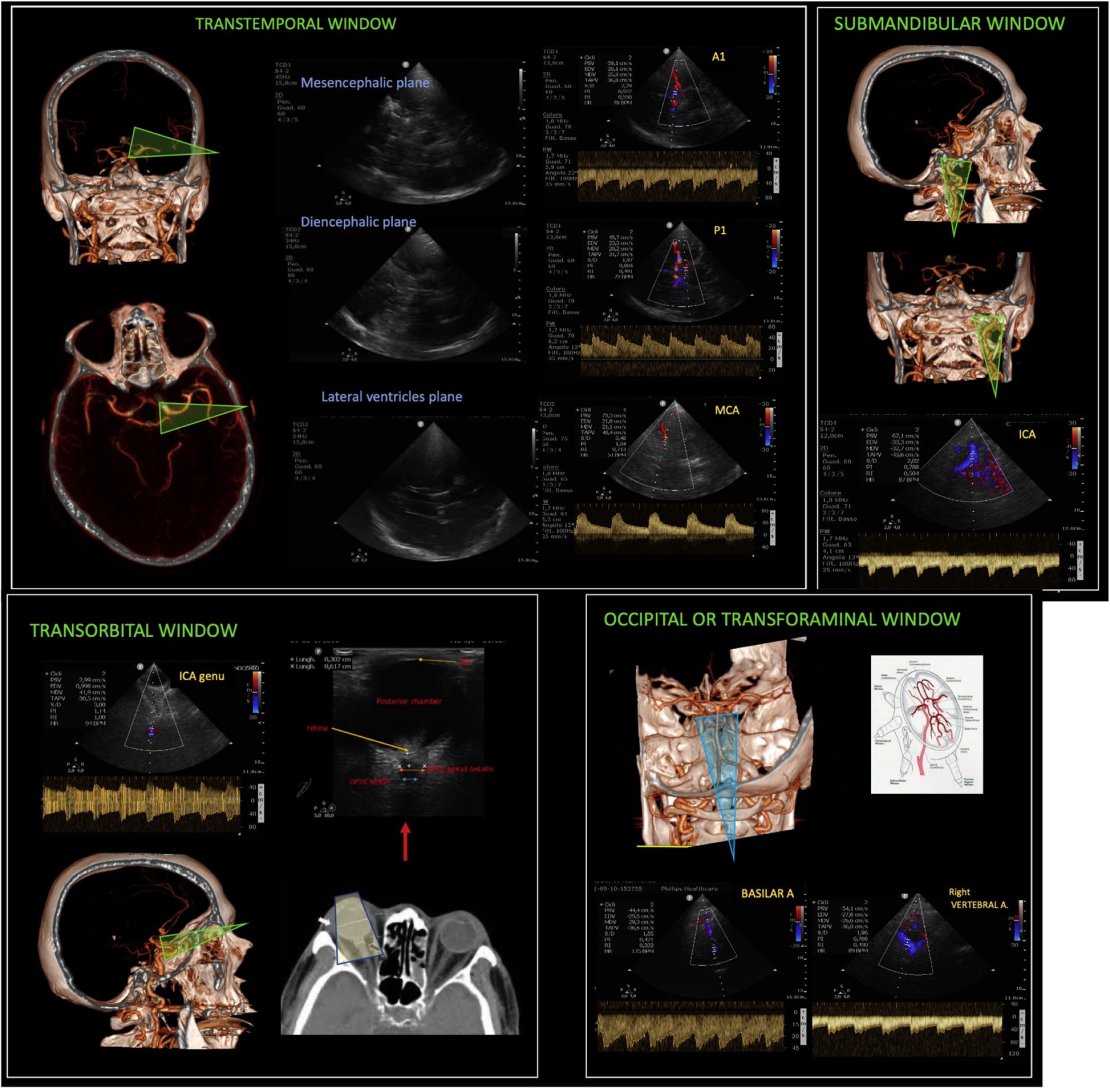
Transcranial color-coded duplex ultrasonography. The insonation windows for cerebral blood flow velocities assessment are shown. For the insonation of the anterior cerebral artery, middle cerebral artery, and posterior cerebral artery, the transtemporal window is used. For internal carotid artery, submandibular window; for basilar artery and vertebral artery, the occipital window is used. A1, anterior cerebral artery A1 tract; MCA, middle cerebral artery; P1, posterior cerebral artery P1 tract; ICA, internal carotid artery
